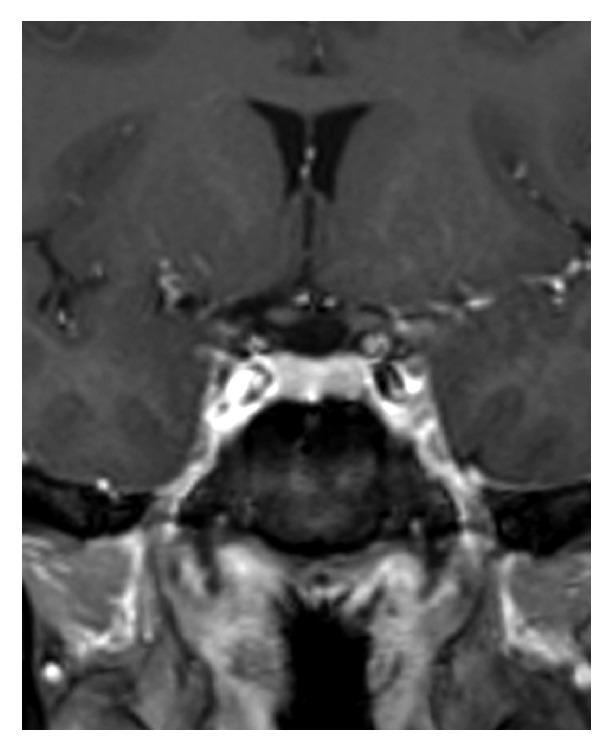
T1-weighted gadolinium-enhanced MRI performed 6 months after surgery showed no evidence of residual tumor.
